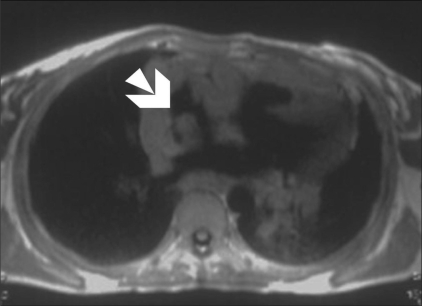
T1W axial MRI image shows diffuse myopericardial thickening, with the thickened myocardium projecting into the right atrium (arrow)
